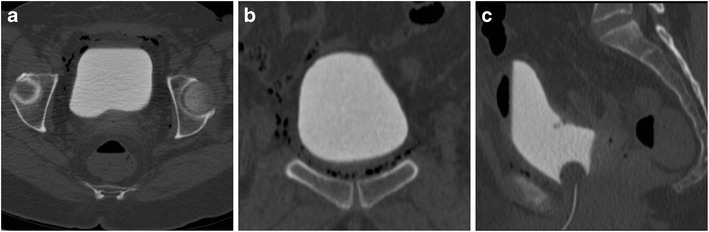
Multidetector CT-cystography performed 4 h after radical surgery for ovarian cystoadenocarcinoma, with intraoperative suspicion of bladder rupture. Multiplanar images show optimal distension of the normal-shaped urinary bladder by diluted contrast medium instilled via the Foley catheter. Note perivesical gas bubbles. The lack of extravasated contrast in the perivesical spaces allowed confident exclusion of either intra- or extraperitoneal bladder injury and vesico-vaginal fistula (Reproduced from open access ref. no. [18])
